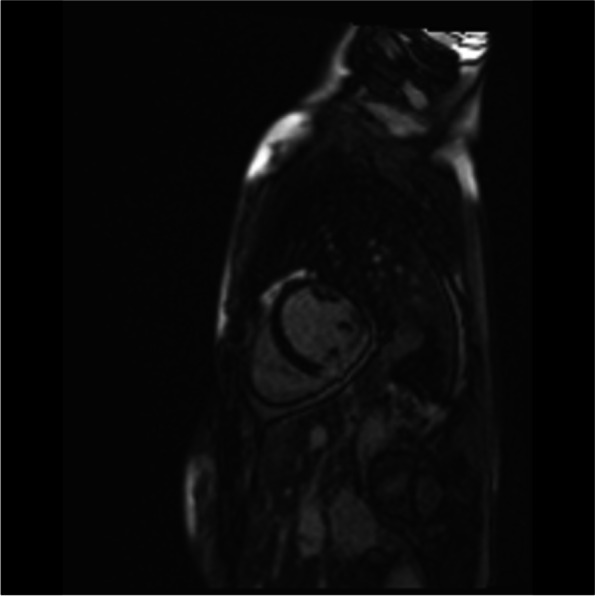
Cardiac MRI inverse recovery LGE sequence, midventricular short-axis view, showing transmural late gadolinium enhancement of the mid inferior segment in an ischemic distribution pattern
